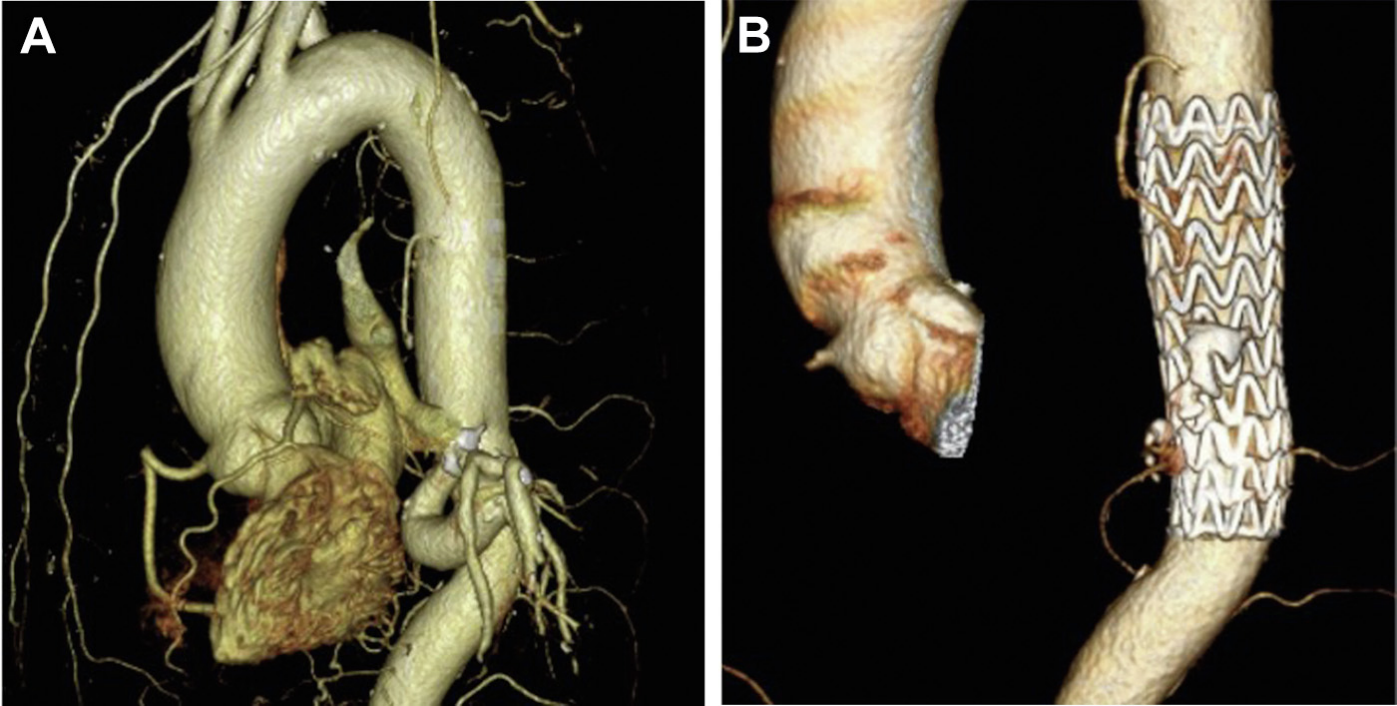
A, Preoperative contrast-enhanced computed tomography image. An enlarged aberrant artery bifurcating from the descending aorta directly into the left lung base area can be seen. B, Contrast-enhanced computed tomography image after thoracic endovascular aortic repair. The aberrant artery can no longer be seen.
